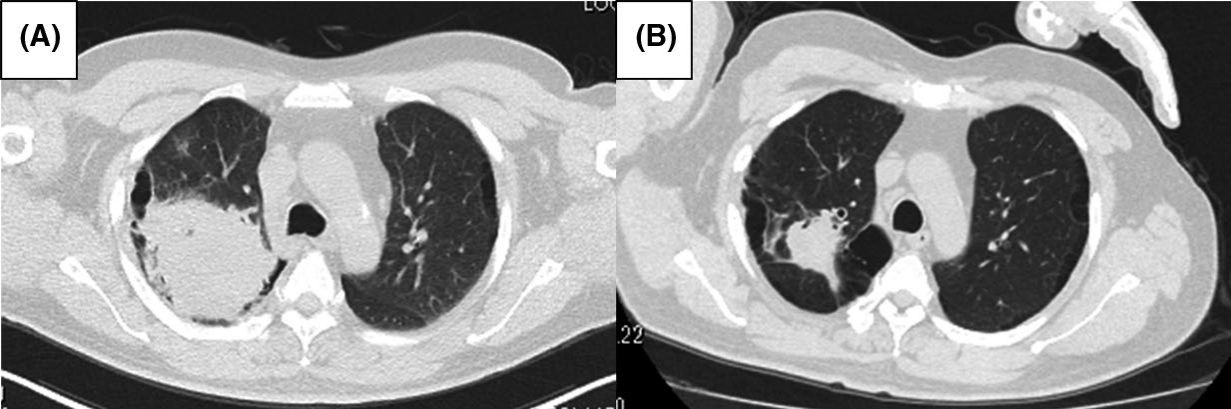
Chest computed tomography showing the primary lesion in the right upper lobe at the time of diagnosis of lung cancer (A) and after four cycles of carboplatin, nab‐paclitaxel, and pembrolizumab and four cycles of pembrolizumab maintenance (B)
